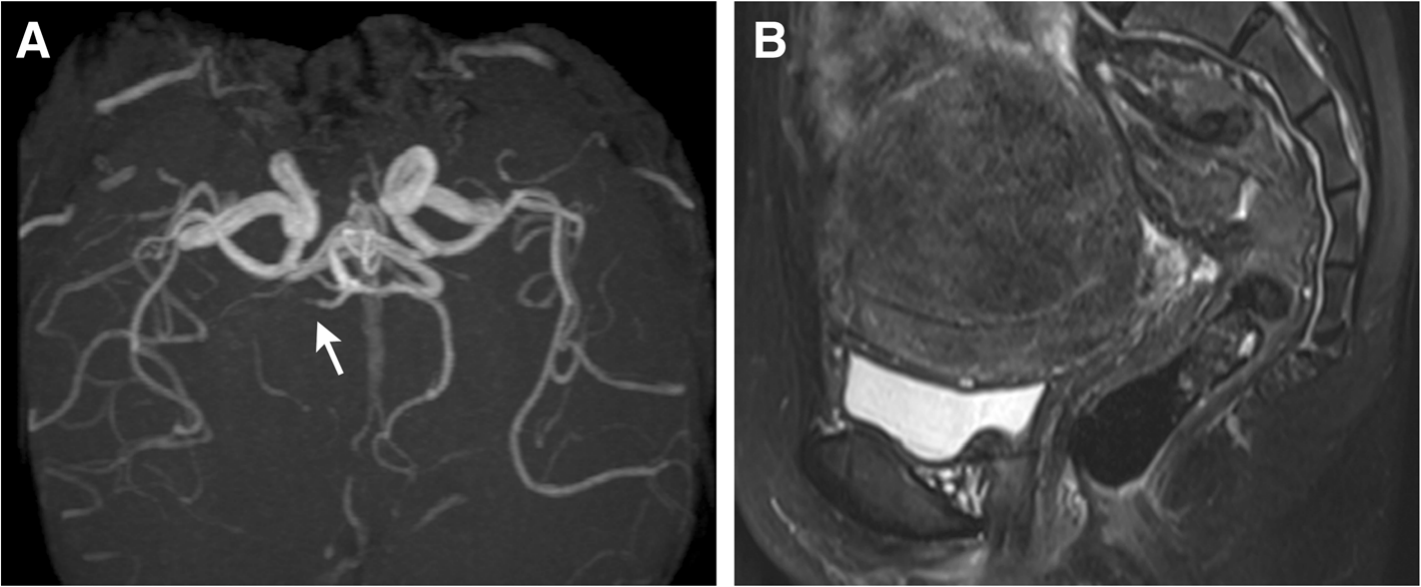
Brain Magnetic resonance angiography (MRA) and pelvic magnetic resonance imaging (MRI) of Case 3. a. MRA shows stenosis of the right posterior cerebral artery. b. Sagittal T2-weighed MRI shows an enlarged uterus with an ill-defined low signal intensity lesion in the posterior myometrium
